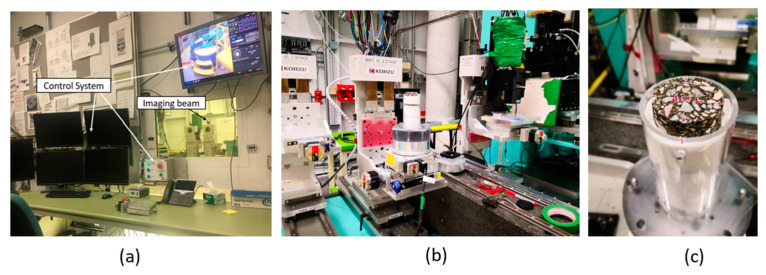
(a) BMIT-ID beamline and controls; (b) AC sample in the BMIT-ID beamline; (c) AC sample inside the mold for the X-ray CT scan.
